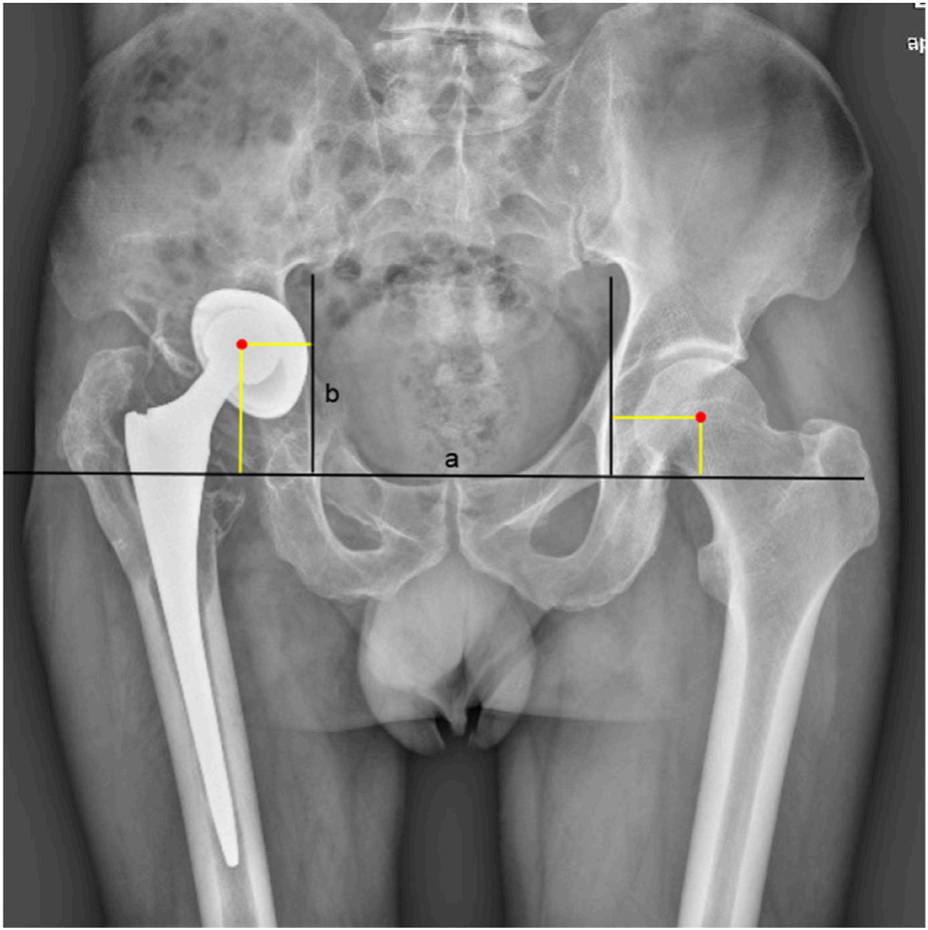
Radiographic Measurement of Hip Center of Rotation (COR). V-COR: Vertical distance between the femoral head center and the radiographic teardrop line (Line a). H-COR: Horizontal distance between the femoral head center and the vertical line through the inferior point of the teardrop (Line b).
